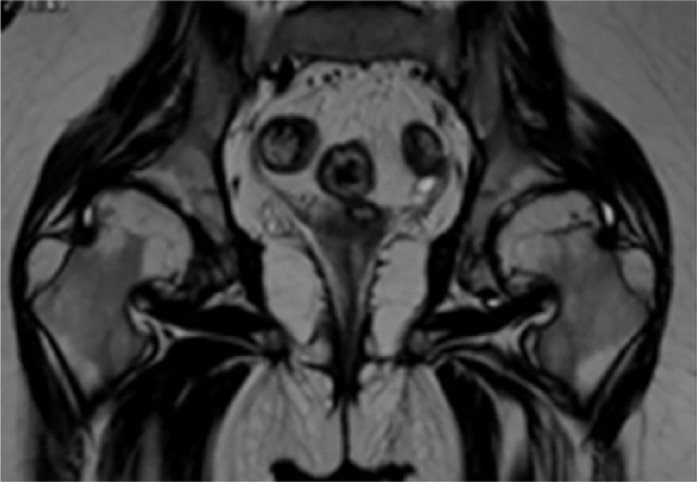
Magnetic resonance imaging (1.5 T) in a 13-year-old girl with PPRC showed intraarticular pathology strongly compatible with the radiographic features of osteoarthritis. Note the laterally prominent femoral head margins create bilateral femoral head asphericity. The capital femoral epiphyses are flattened, irregular associated with metaphyseal fragmentation and irregularity. The superior portions of the acetabular labra were sclerosed with partial detachment.
